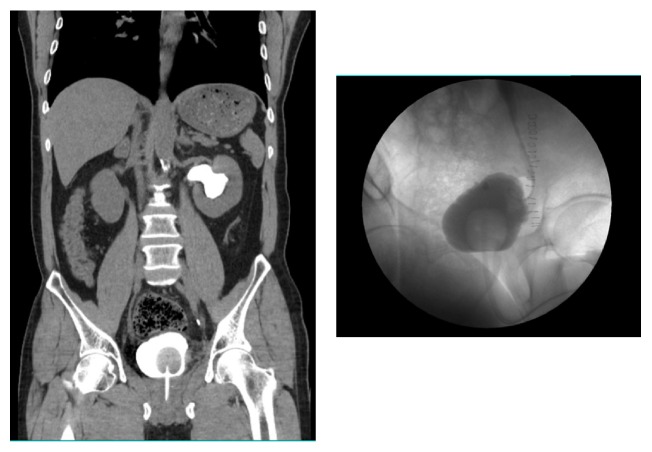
Computed tomography showing (a) abnormal elevation of the left ureterovesical area of the bladder with hydronephroureterosis and (b) degree of hydronephroureterosis with kinking ureter.
